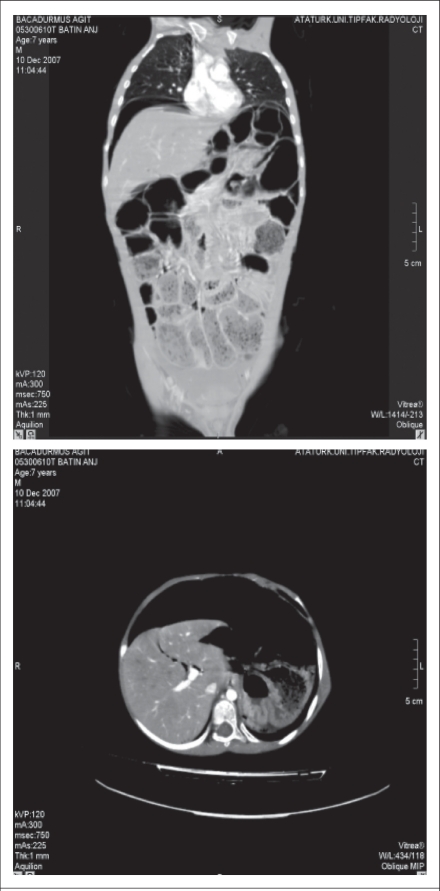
Abdominal CT before treatment
